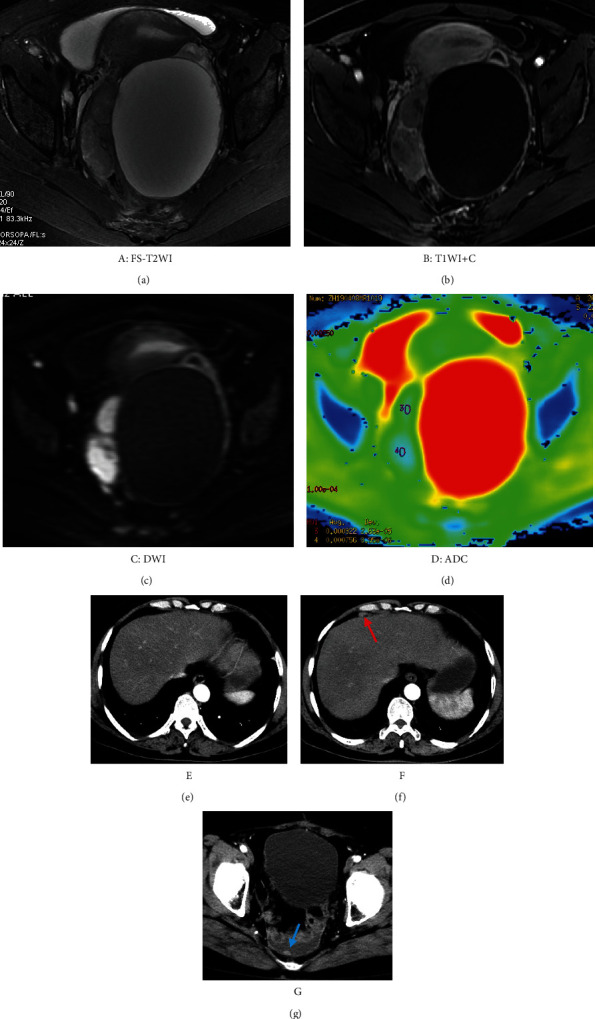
(a–d) A 54-year-old female was diagnosed with bilateral ovarian high-grade serous carcinoma (HGSOC, FIGO IIIC) by postoperative pathology on April 18, 2019. Postoperative chemotherapy was performed six times with TC regimen [docetaxel (75 mg/m^2^) + carboplatin (AUC = 5)]. (e) Reexamination of chest+abdominal CT and pelvic MRI on January 20, 2020. No clear recurrent diseases were found. (f, g) On April 25, 2021, the CA125 was elevated, and CT examination revealed thickening of the abdominal peritoneum with multiple nodules (red and blue arrow) and pelvic effusion, so recurrence was considered. DFS was 24 months.
